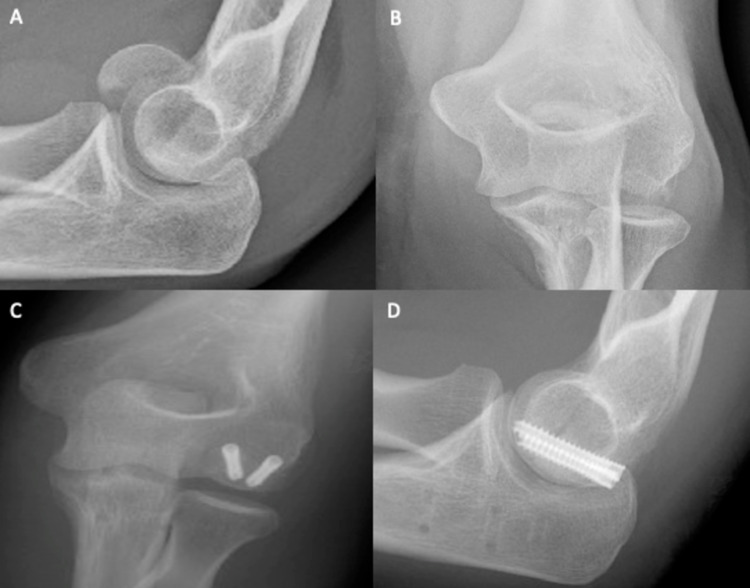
Plain elbow X-rays of case 4. Figure 5A, 5B shows anteroposterior and lateral views, respectively, of a type I capitellum fracture. Figure 5C, 5D shows anteroposterior and lateral views, respectively, of the elbow after ORIF. ORIF: open reduction and internal fixation
